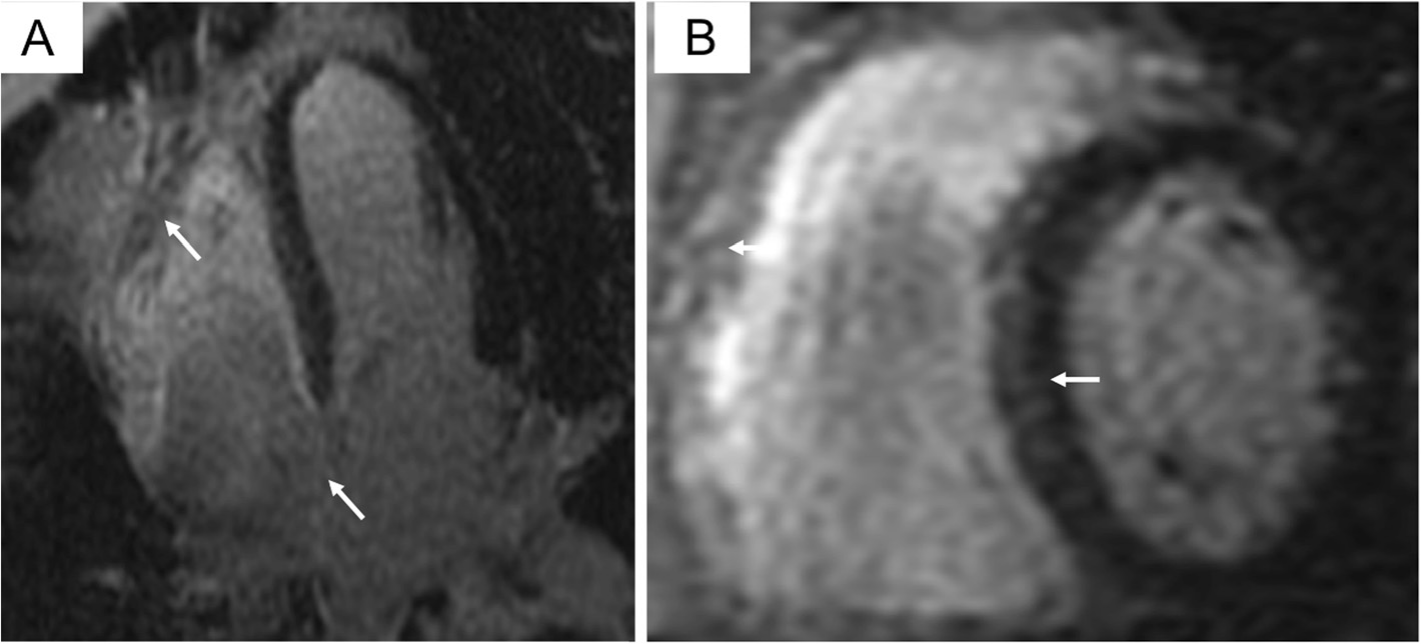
Delayed enhancement cardiac magnetic resonance images in the four-chamber (A) and short axis (B) image plane. The images show a patchy pattern of delayed enhancement (arrows) in the subepicardial and mid-wall of the right ventricle free wall, interventricular and interatrial septum. The myocardium of the left ventricle free wall is normal.
